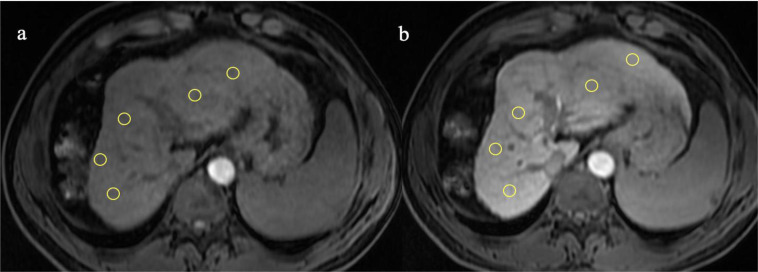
Regions of interest (ROI) placement for liver enhancement ratio measurement on gadoxetic acid-enhanced magnetic resonance imaging. Circular ROIs were placed in identical locations on both precontrast and hepatobiliary-phase images, covering both hepatic lobes and avoiding vascular and biliary structures.
